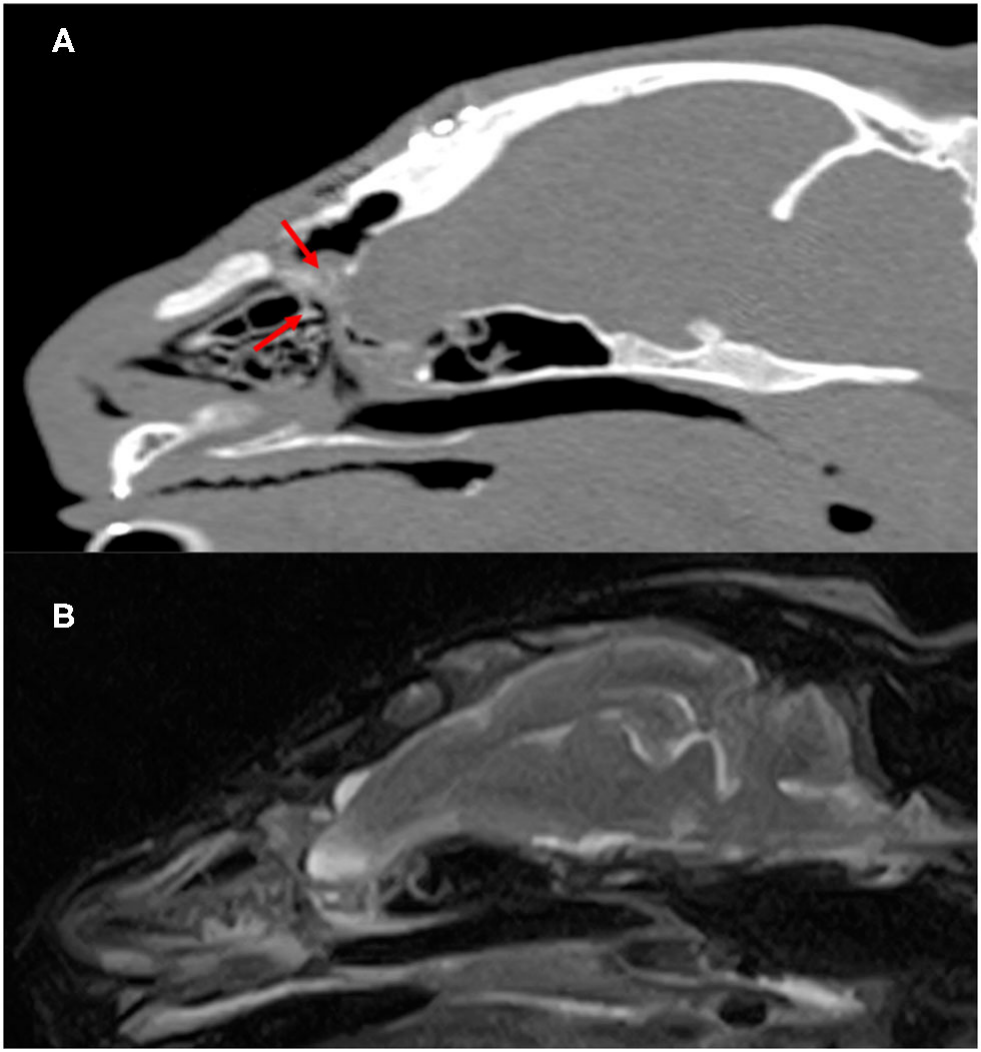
Postoperatve CT and MR images of the cranium and brain 7 months after surgery. Parasagittal CT images (A) showed slightly superabsorbent structures separate cranial cavity and nasal cavity (red arrow). On parasagittal T2-weighted MR images (B), there is no apparent departure of the brain into the nasal cavity.
